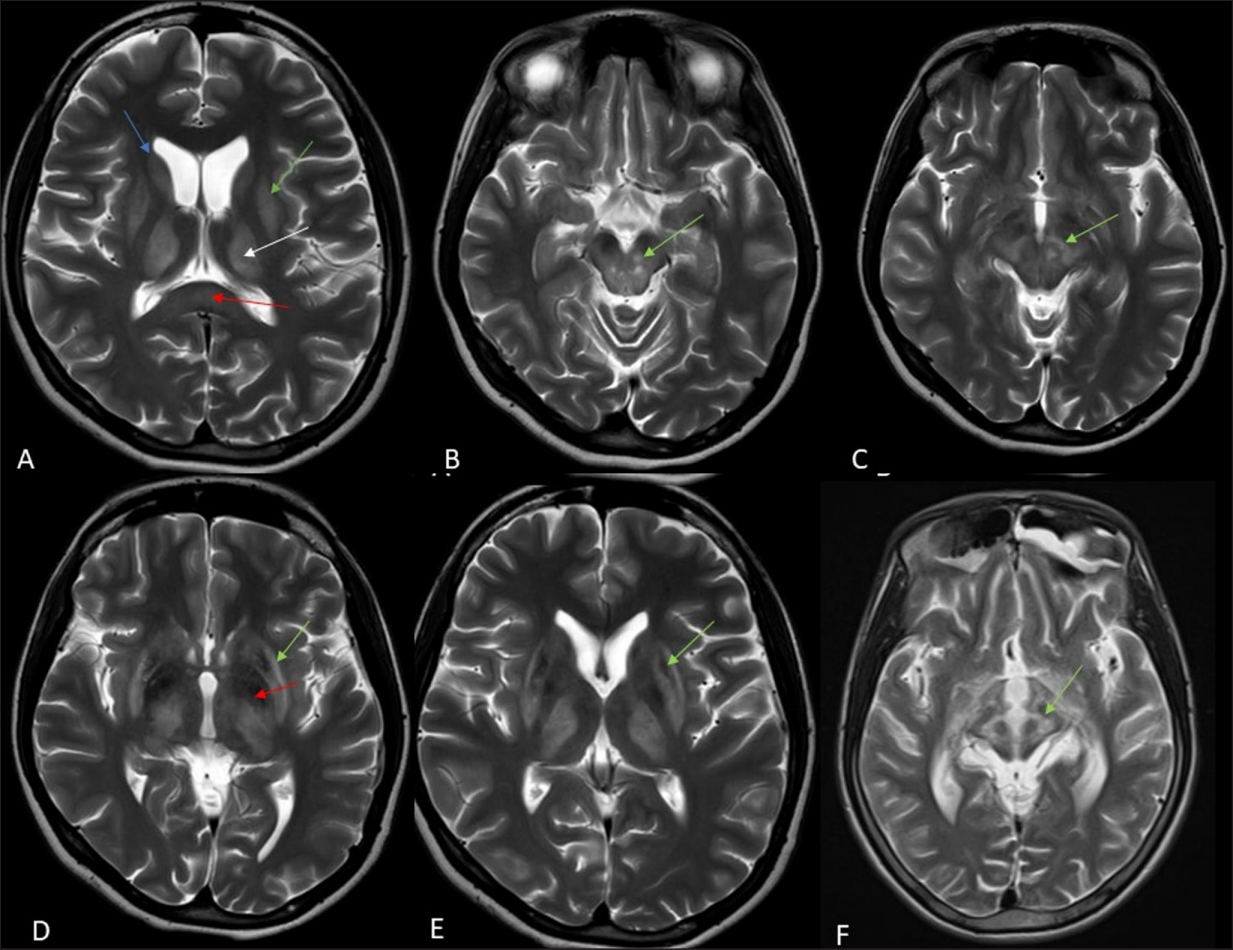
Brain MRI axial T2-weighted images showing hyperintense lesions in (A) bilateral caudate (blue arrow), putamen (green arrow), thalamus (white arrow) and splenium of corpus callosum (red arrow); (B) and (C) midbrain tegmentum (green arrow); (D) ‘Bright claustrum’ sign (green arrow), hypointense lesions in bilateral globus pallidum (red arrow); (E) central T2-hypointense lesion surrounded by hyperintensity in bilateral putamen (green arrow); (F) ‘Face of giant panda’ sign in midbrain (green arrow). MRI: Magnetic resonance imaging.
